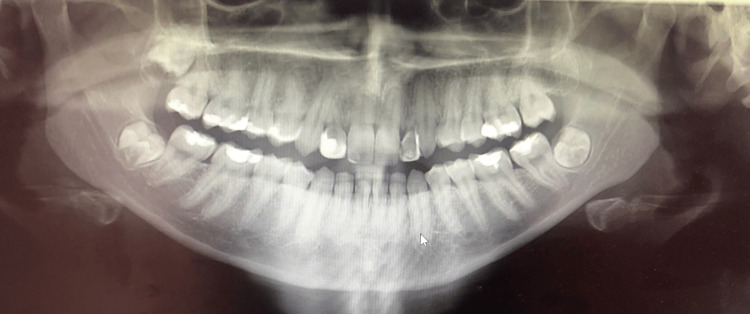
Radiograph - OPG OPG, Orthopantomogram
